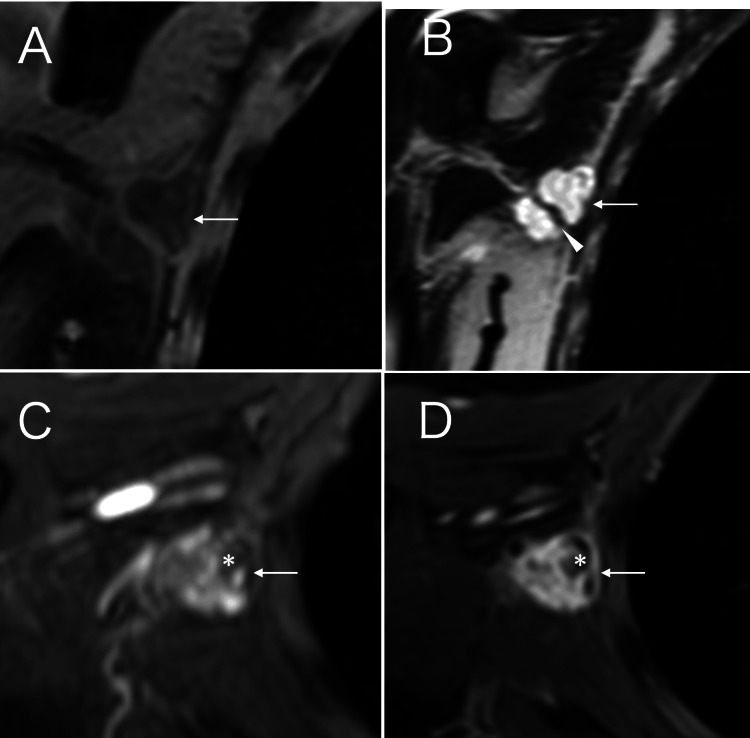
MRI findings A. MRI of the tumor (arrow) showed slightly high signals on fat-suppressed T1-weighted images. B. MRI of the tumor (arrow) showed strong high signals with linear low signals corresponding to the intra-tumoral septum (arrowhead) on T2-weighted images. C. MRI of the tumor (arrow) showed enhancement with focal non-enhanced areas (asterisk) on early phase dynamic studies. D. MRI of the tumor (arrow) showed stronger enhancement with retained no enhanced areas (asterisk) on late phase dynamic studies MRI: magnetic resonance imaging
